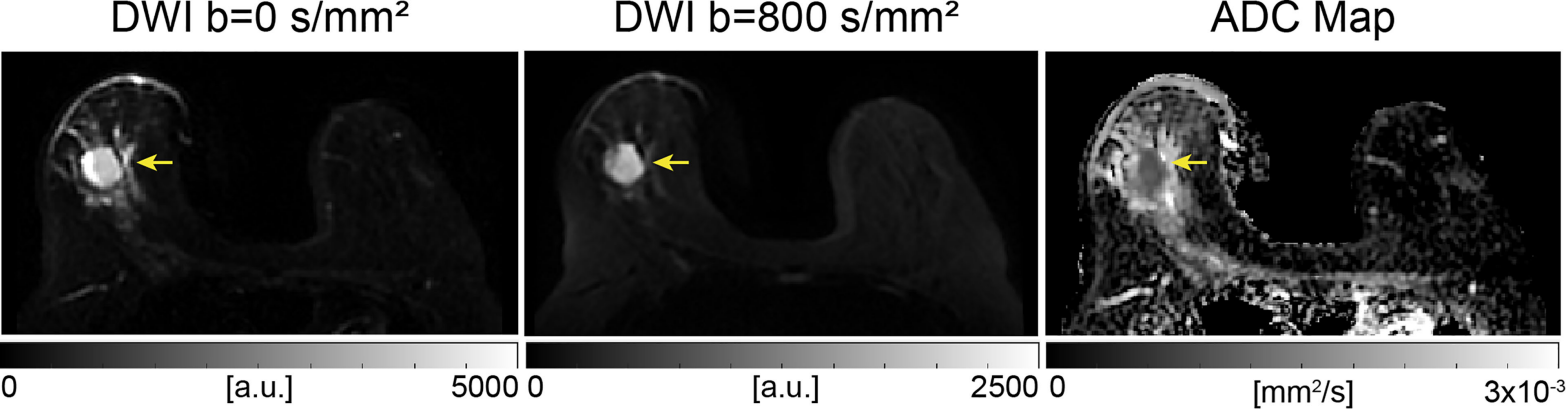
Example of conventional breast DWI at 3T, shown at b-values 0 and 800 s/mm^2^ and corresponding ADC map in a 49-year-old patient The lesion, indicated by the yellow arrow, has increased signal on b=800 s/mm^2^ images and displays lower ADC values compared to surrounding tissue, indicating a finding suspicious for malignancy. This lesion was found to be an invasive ductal carcinoma from pathology.
